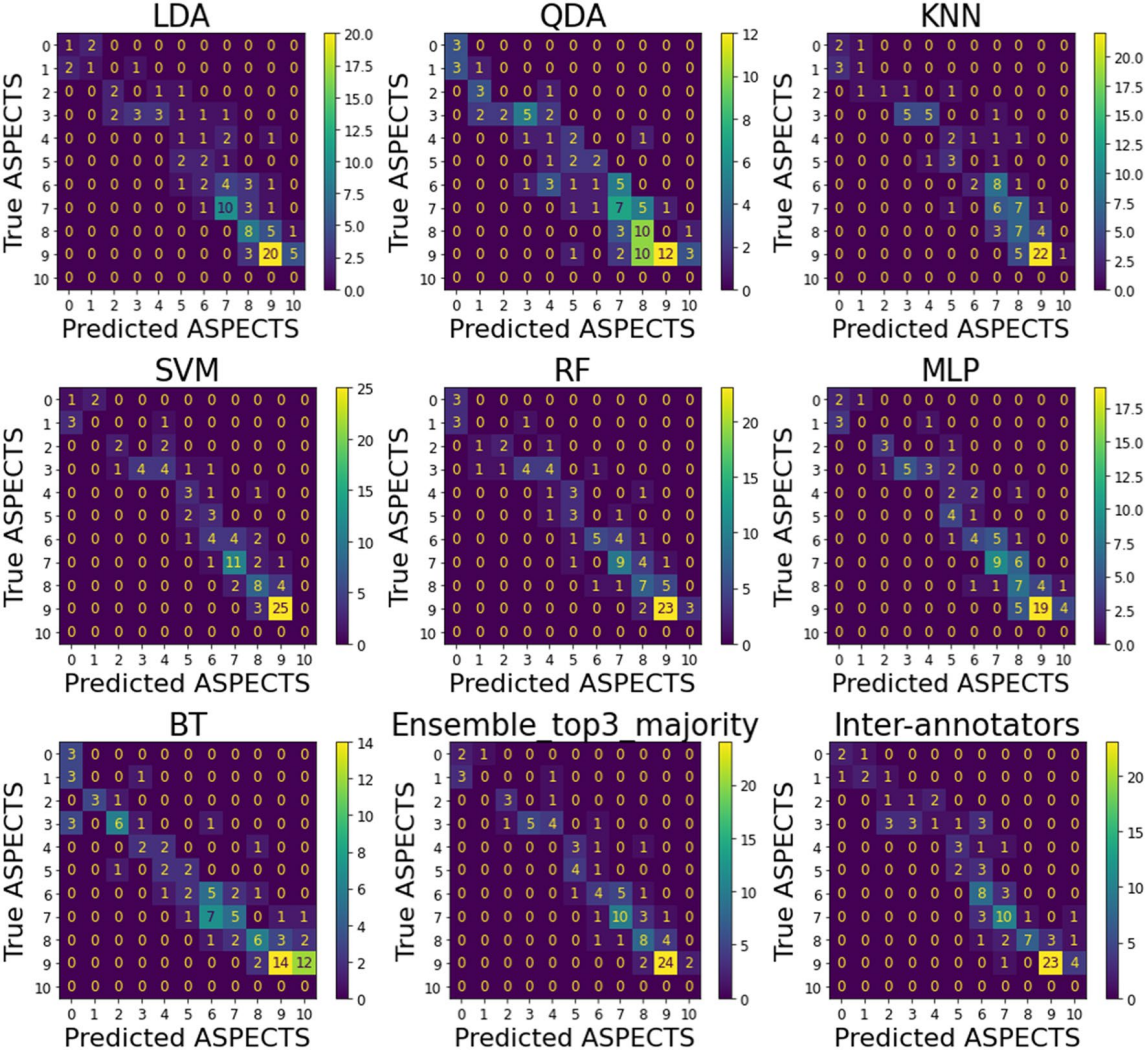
Confusion matrices of ML models in the external testing set (n = 100). A potential perfect model would result in zeros outside the diagonal (i.e., no prediction errors). The cells adjacent to the diagonal represent "acceptable" errors (predicted ASPECTS within ± 1 difference from the ground true ASPECTS).
